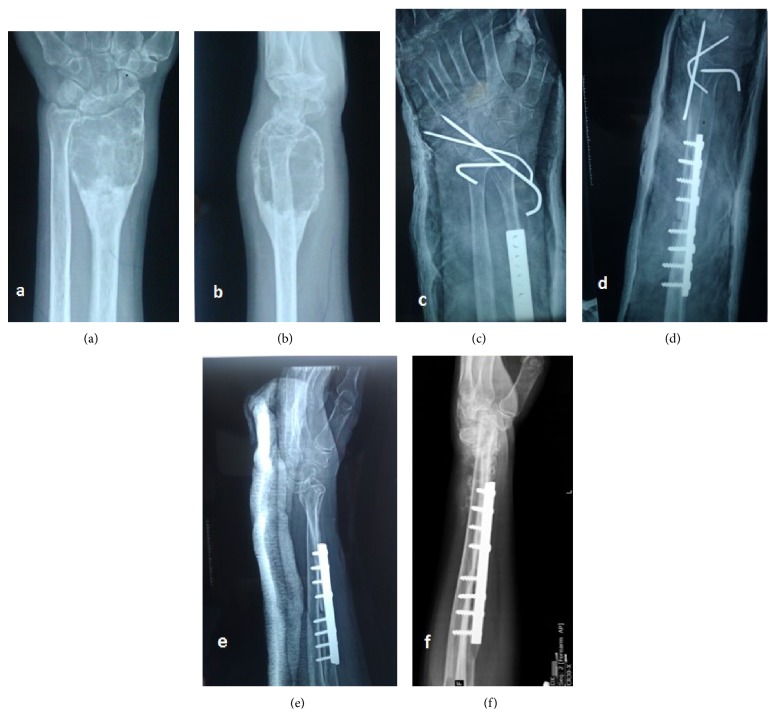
(a and b) Preop plain radiograph of left wrist showing expansile solitary lytic lesion involving left distal radius; (c and d) postoperative radiographs after resection and reconstruction arthroplasty of left wrist; (e) radiographs of her left wrist showing wrist subluxation following k-wire removal; (f) 1 year follow-up radiograph of her left wrist with gross subluxation of wrist.
